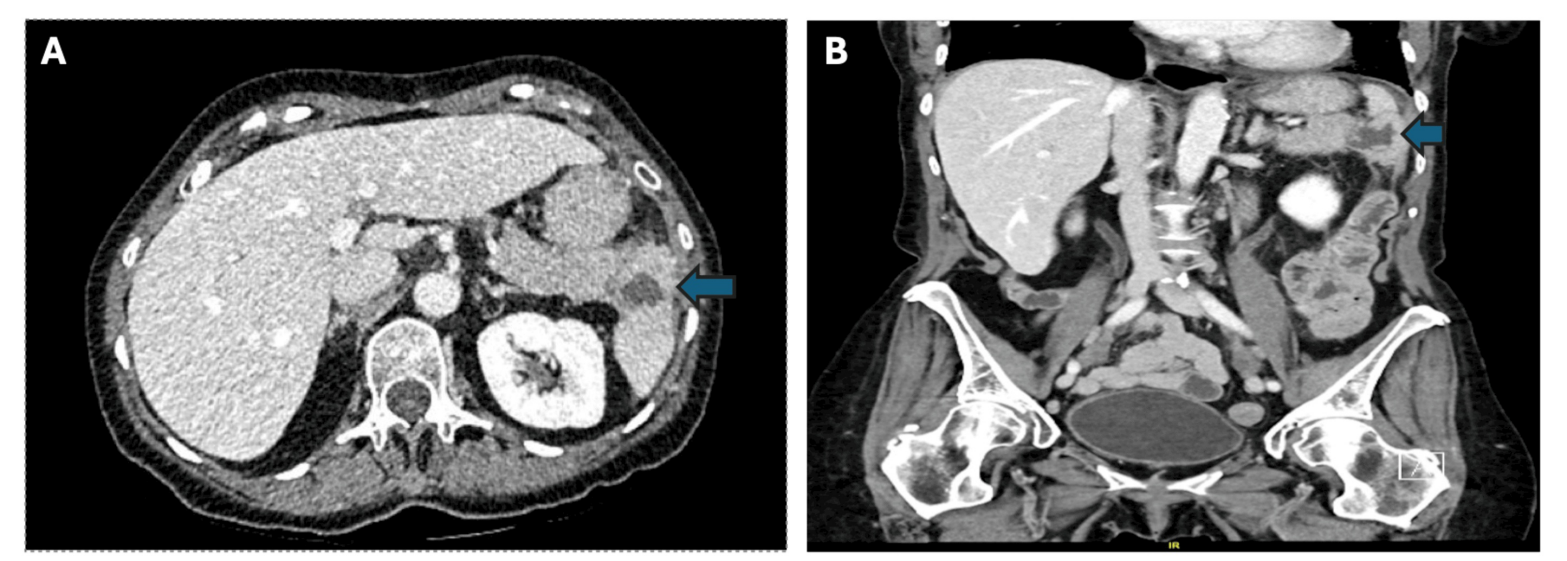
CT of the abdomen and pelvis CT of the abdomen and pelvis with contrast showing initial splenic fluid collection in the axial (A) and coronal (B)views. Fluid collection is denoted by blue arrows
